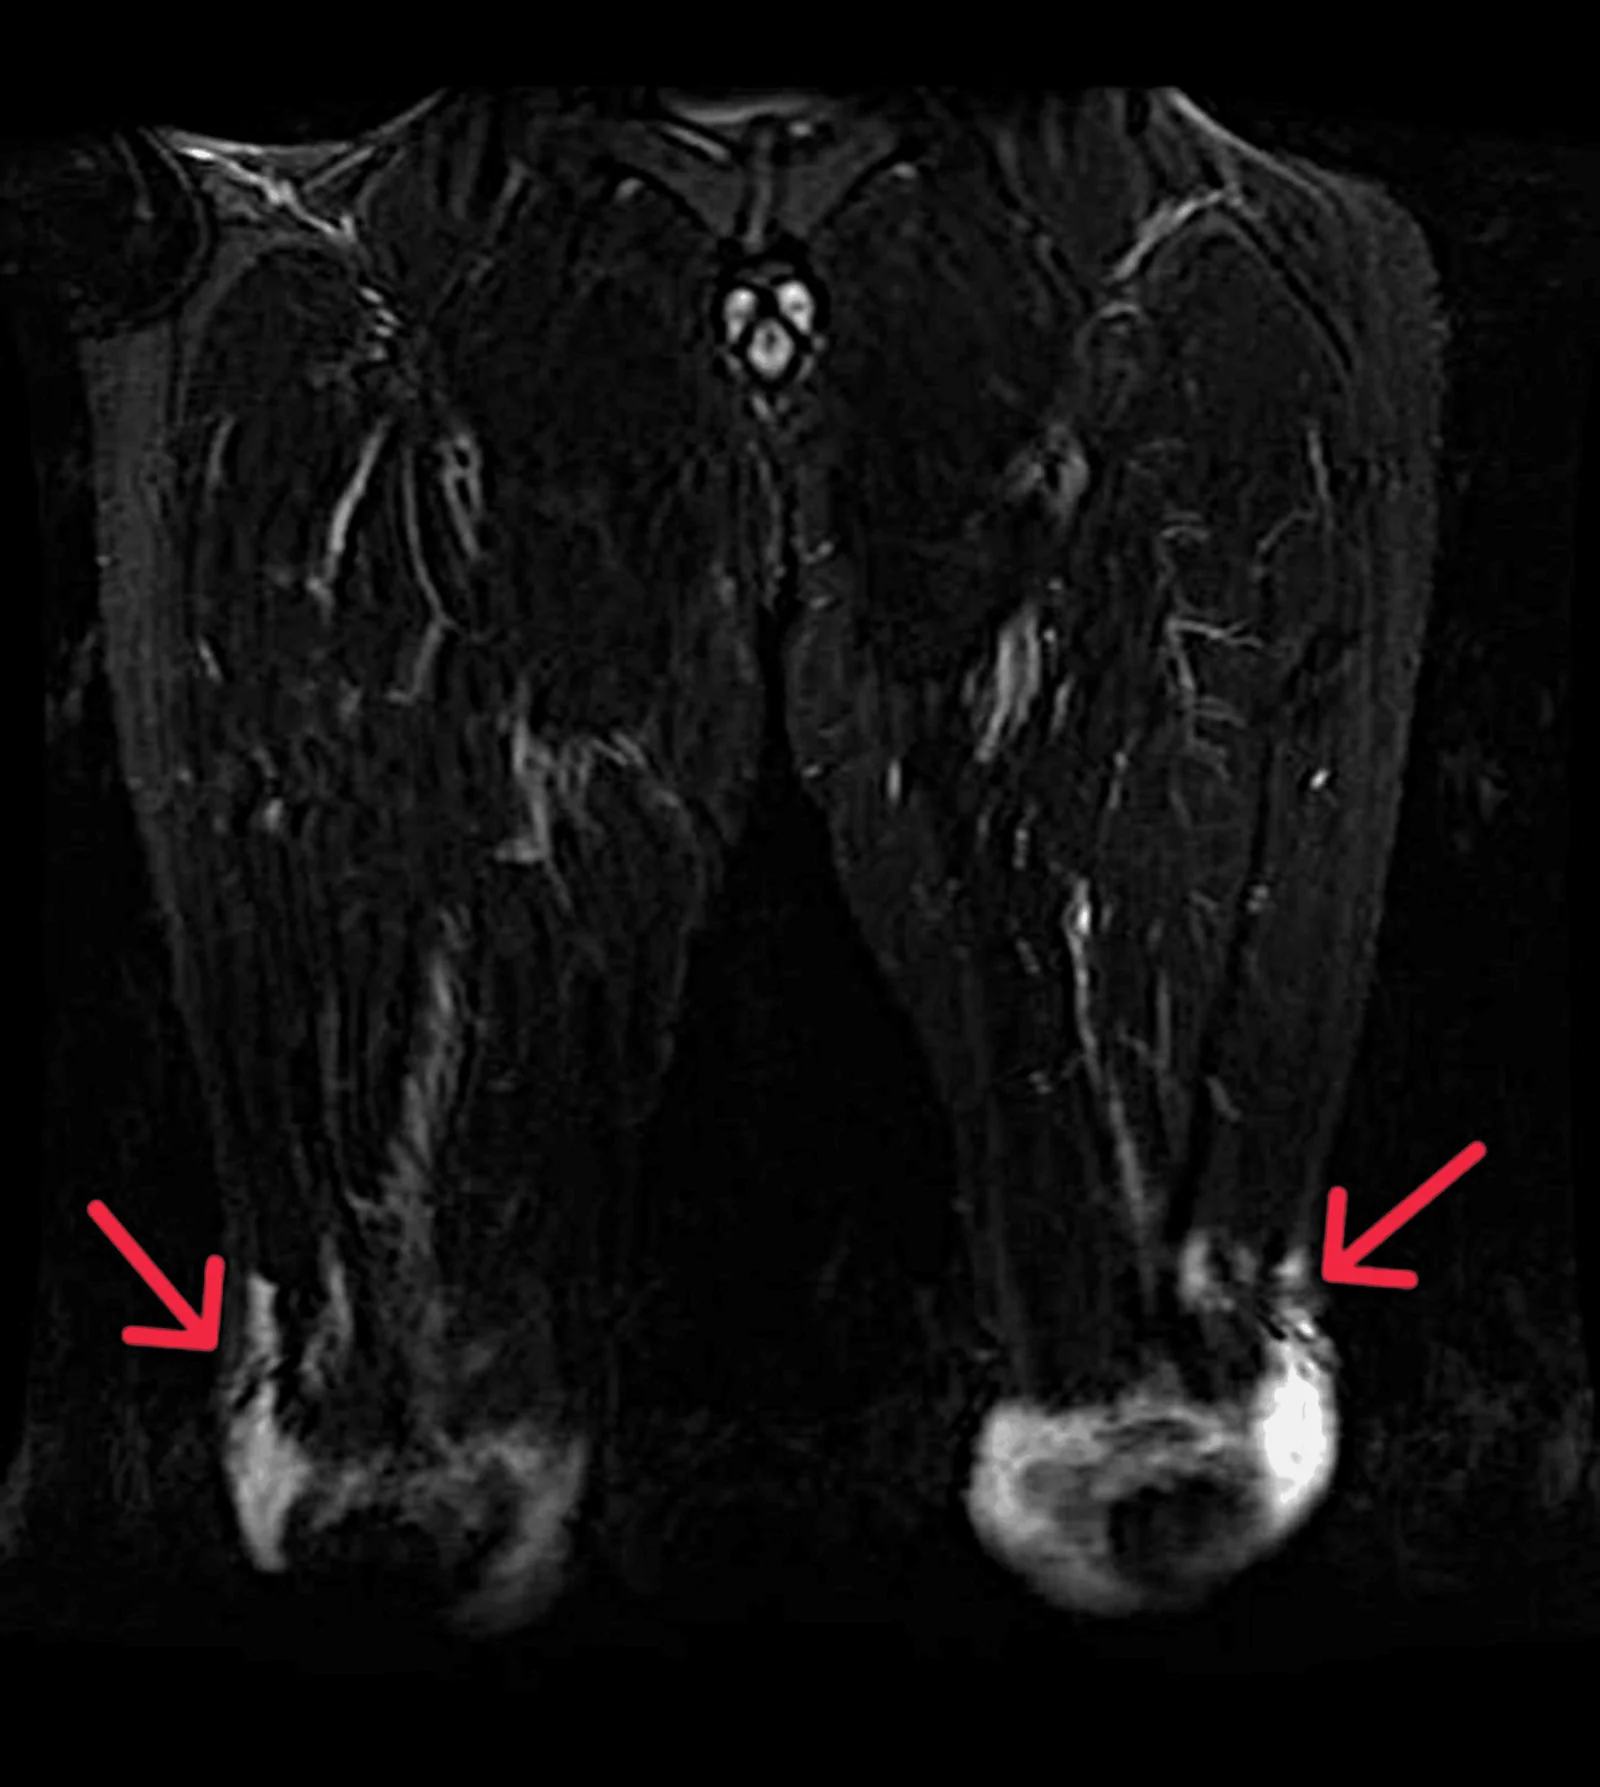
Coronal T2-weighted MRI of both knees demonstrating bilateral complete quadriceps tendon ruptures at the superior poles of the patellae, with tendon retraction and surrounding soft-tissue edema (arrows).
